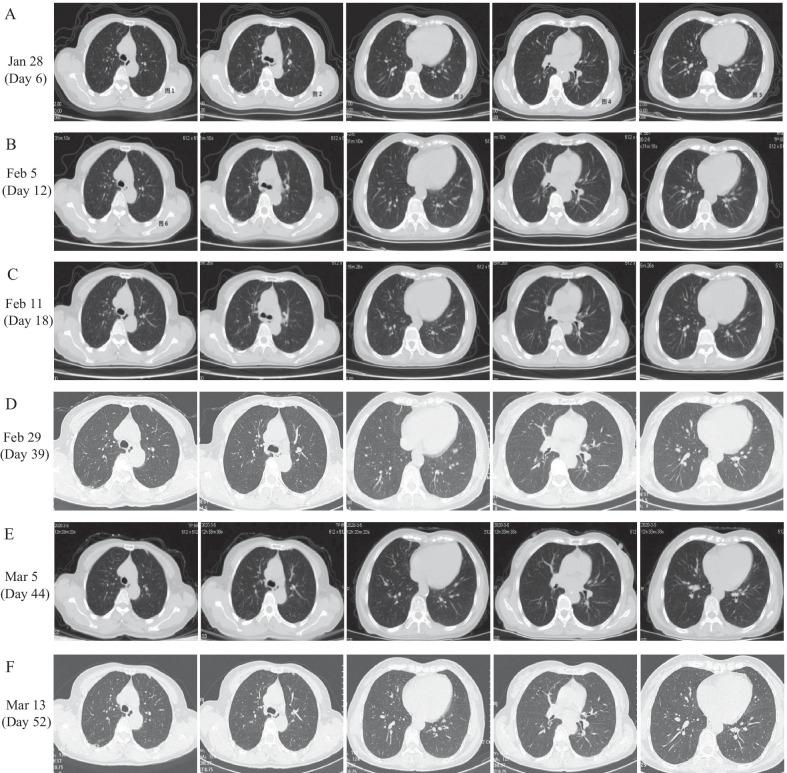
CT of the chest obtained on different time points of the disease course
